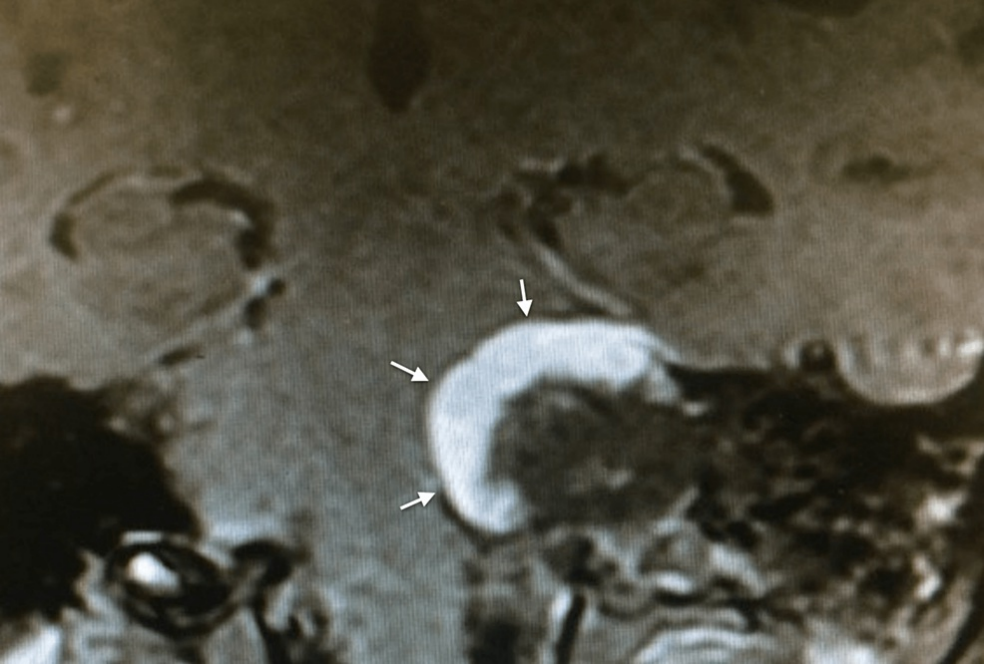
Postoperative coronal contrast-enhanced T1-weighted MRI showed subtotal resection (white arrows)
